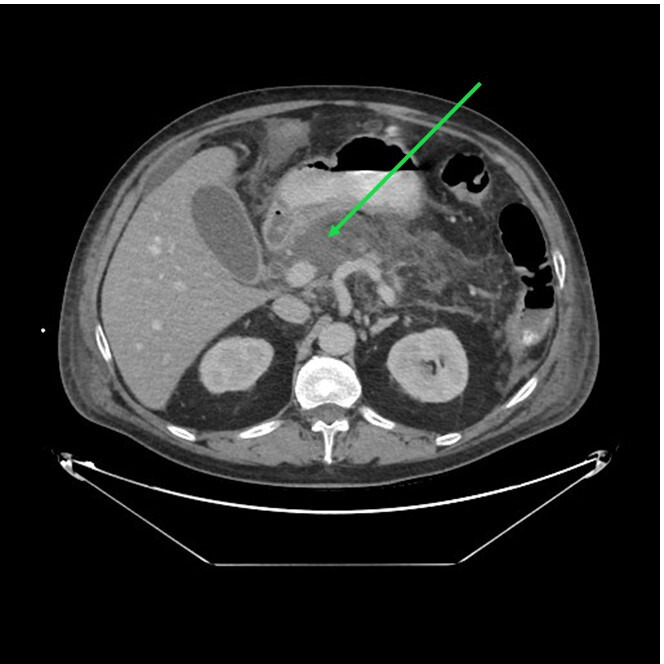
CT scan revealing hypodense areas corresponding to pancreatic necrosis (green arrow).
